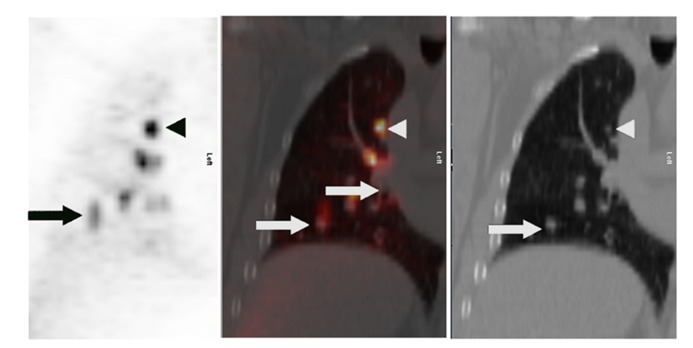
Lung cancer patient with multiple right lung nodules. Shown are corresponding PET (*left*), CT (*right*), and fusion (*middle*) images of a single coronal plane through the right lung. In the upper lobe, where respiratory motion is least, a punctate nodule (white arrowheads) demonstrates intense FDG uptake with a distinctly focal appearance (black arrowhead). In the lower region of the lung, where respiratory motion is greater, the FDG uptake of larger nodules (white arrows) appears relatively less-intense – probably due to “spreading” of the activity over a spatial volume during each breathing cycle, a kind of respiratory artifact. This “spreading” is most visually evident closest to the diaphragm (black arrow).
